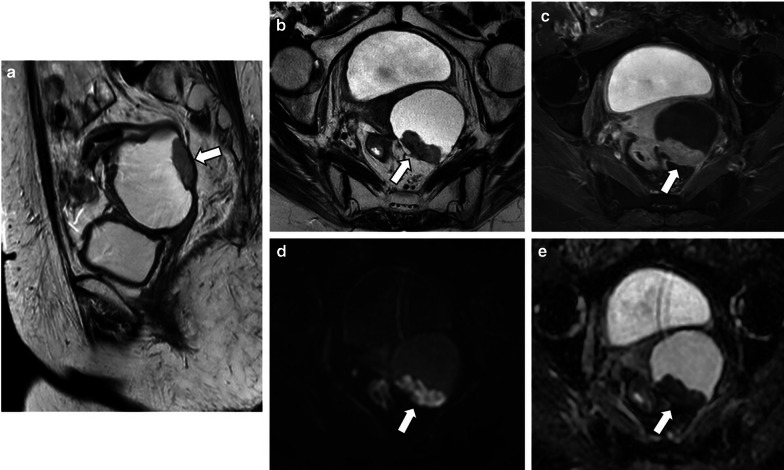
Bilateral LGSC in a 58-year-old female. Sagittal and axial T2-weighted MR images (a, b) and axial T1-weighted MR image with fat saturation, after gadolinium administration (c) demonstrate a left cystic adnexal tumour with solid parietal components (arrows). Axial *b*-1000 s/mm^2^ diffusion-weighted image (d) and ADC map (e) show diffusion restriction in the parietal nodule (arrows)
